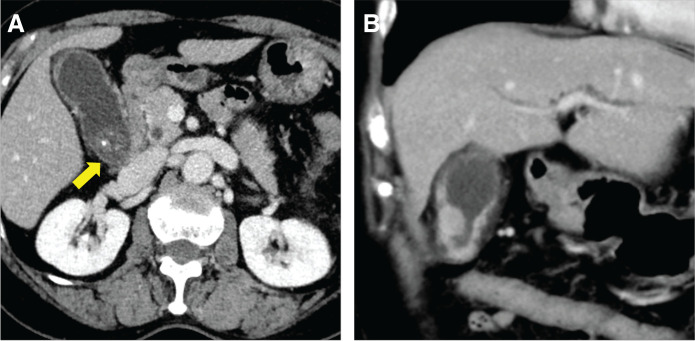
Computed tomography findings. (A) Multiple incarcerated gallstones (yellow arrow) are observed. (B) Swelling of the gallbladder and edematous circumferential thickening of the gallbladder wall are observed.
